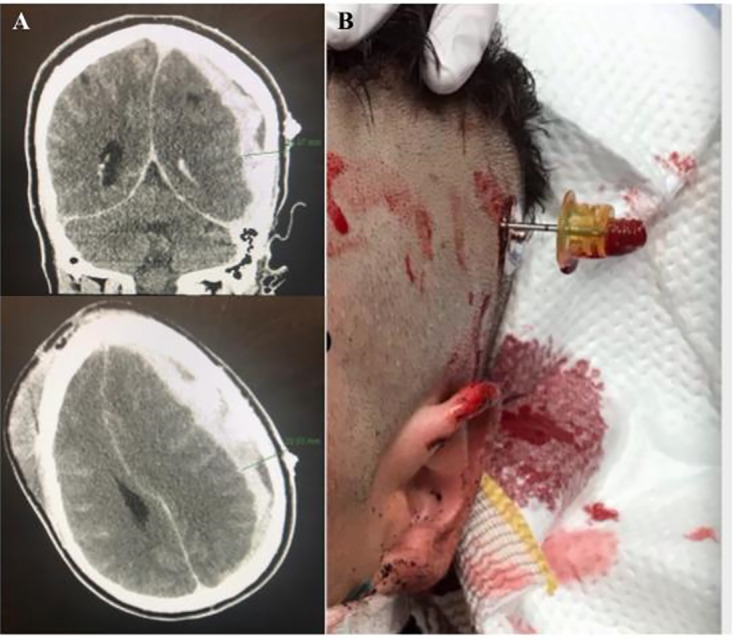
Intraosseous needle positioned on scalp (B) placed just inferior and anterior to marker seen on computed tomography (A) with maximal depth of hematoma measured to guide advancement of needle.
